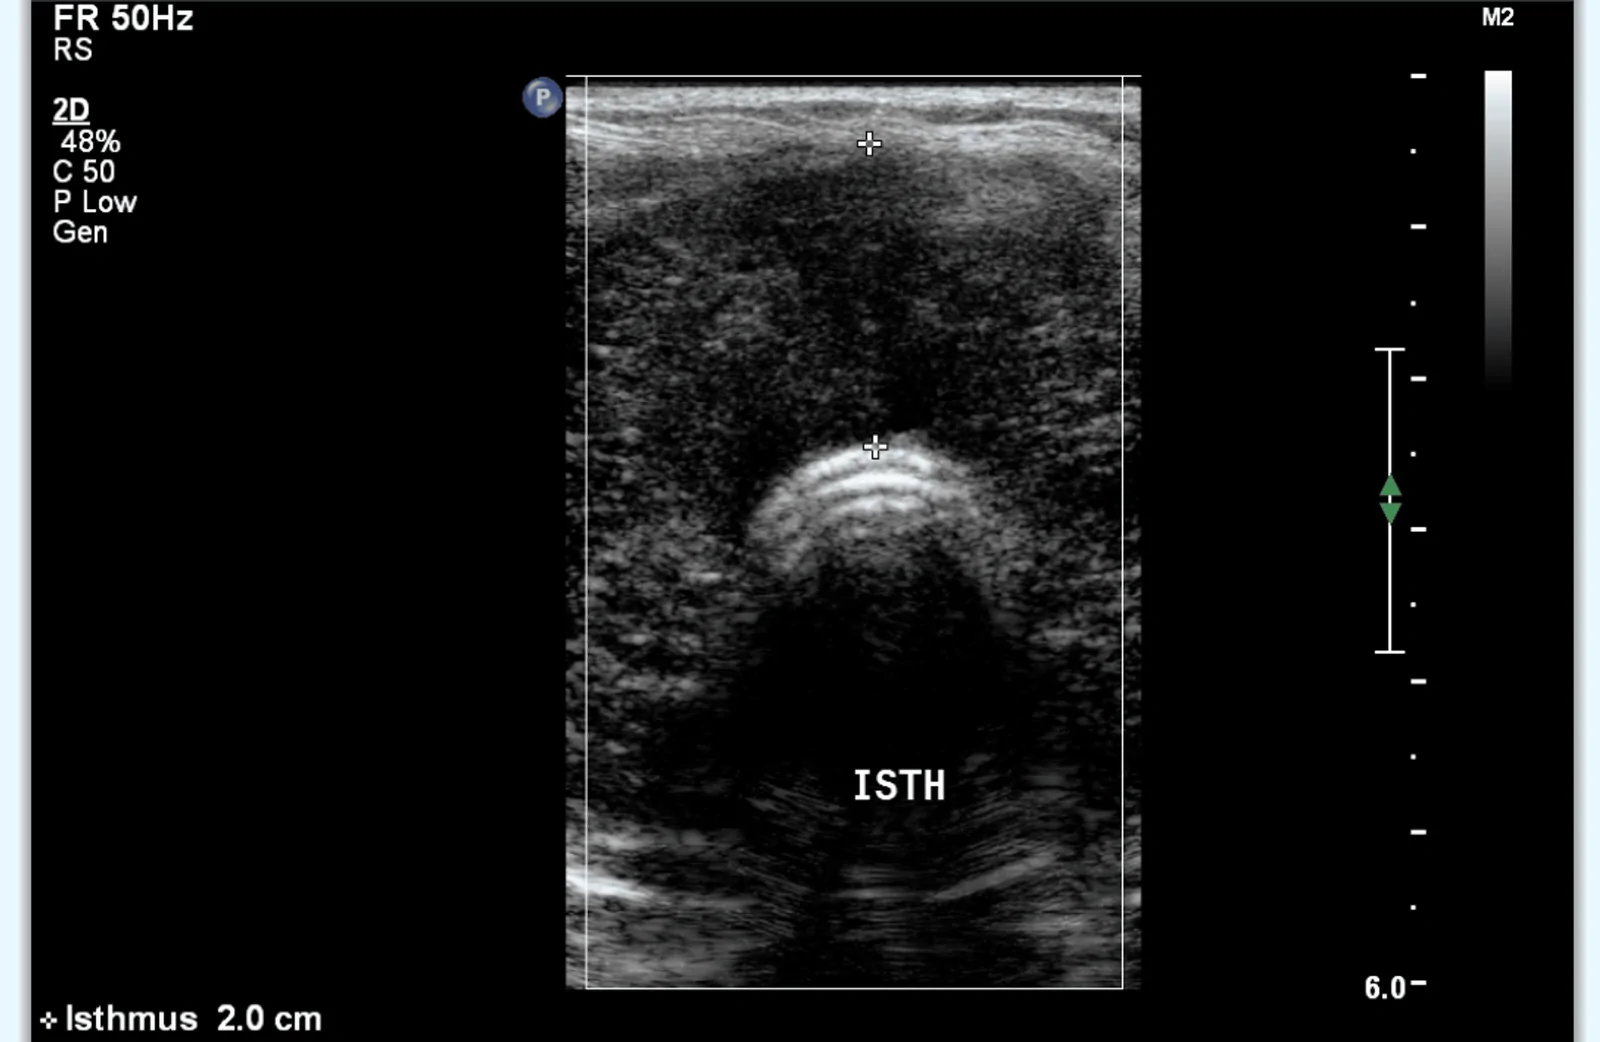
Transverse ultrasound view of the thyroid isthmus, measuring 2.0 cm.
